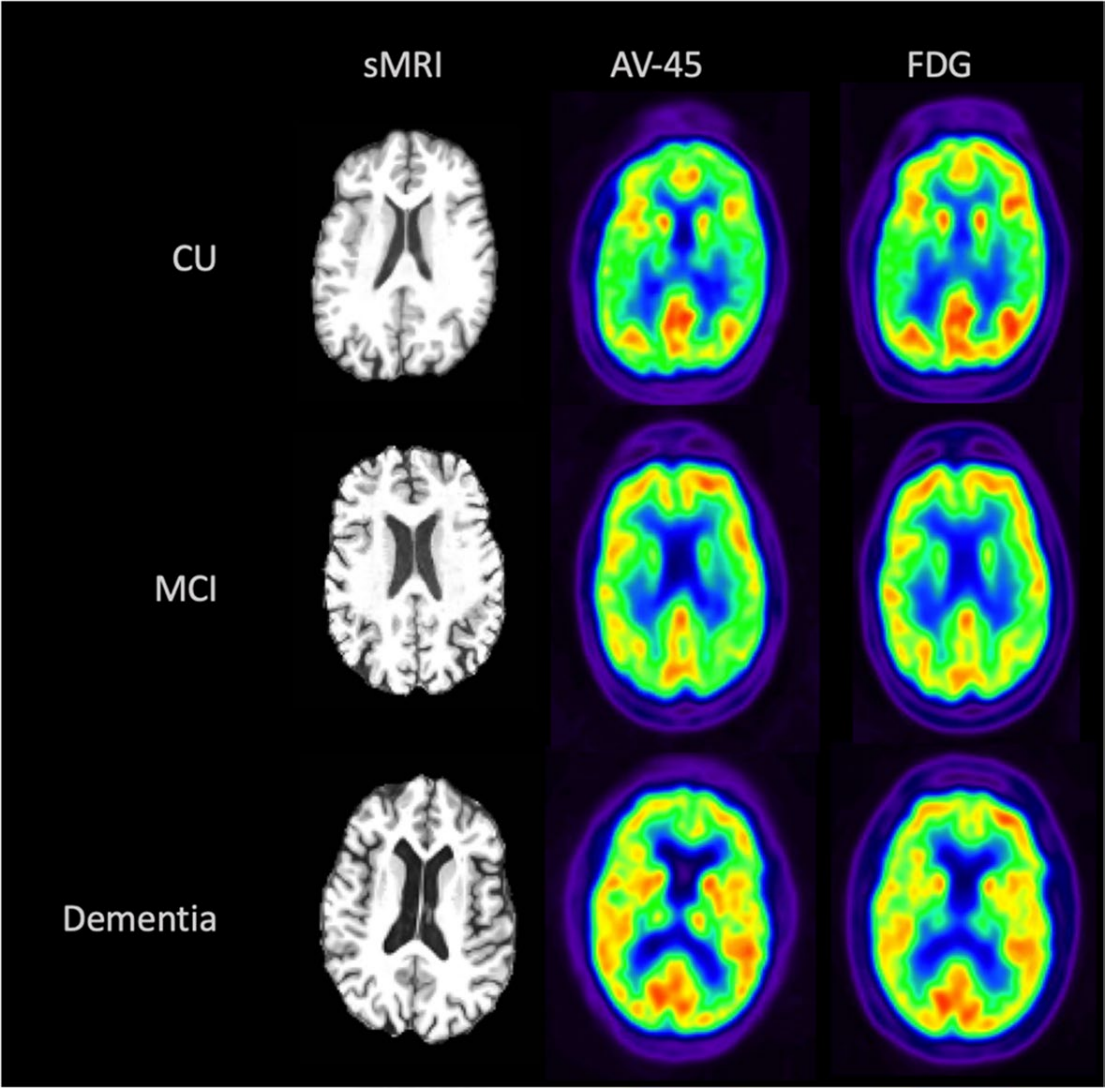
Visual comparison of sMRI, early-frame 18F-florbetapir, and ^18^F-FDG PET for participants from each disease state (CU, MCI, dementia)
